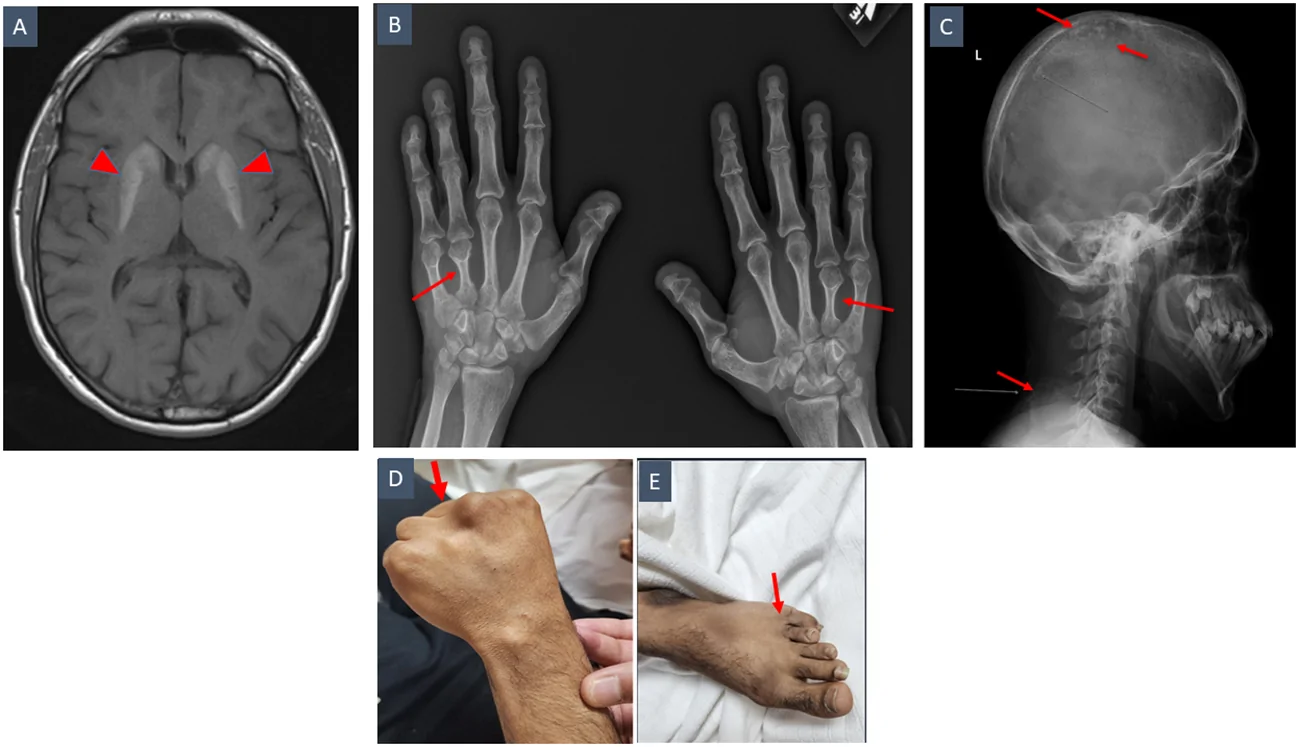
Axial T1-weighted brain MRI demonstrating symmetric hyperintense signal (arrowheads) in the bilateral basal ganglia (A); hand radiographs showing bilateral shortening of the fourth and fifth metacarpal bones (arrows) (B); lateral skull/neck radiograph showing intracranial and cervical soft-tissue calcifications (arrows) (C); clenched left fist demonstrating the characteristic knuckle dimpling caused by shortening of the fourth and fifth metacarpal bones (arrows) (D); and photograph of the left foot demonstrating shortening of the fourth and fifth metatarsal bones (arrows) (E).
